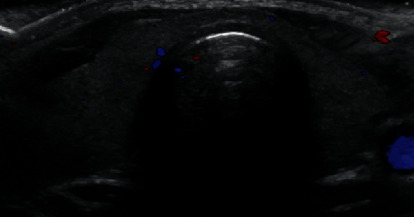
Thyroid ultrasound on post contrast day 7 reveals homogeneous and non enlarged thyroid gland measuring Isthmus: 0.2 cm, Right lobe: 2.3 × 4.3 × 0.9 cm Left lobe: 1.3 × 4.3 × 1.1 cm. Echogenicity is within normal limits with no increased vascularity.
